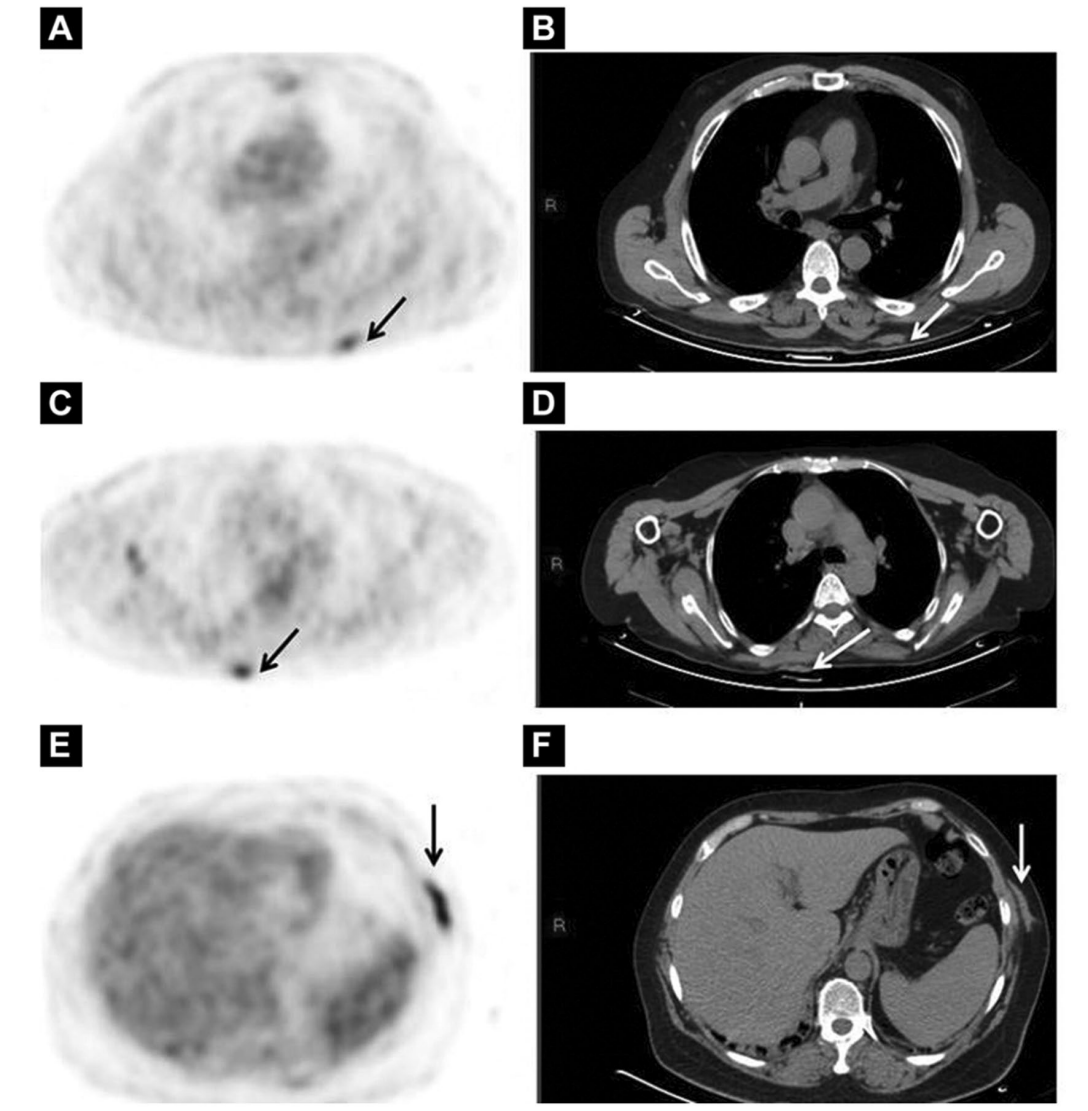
A patient with cutaneous marginal zone lymphoma, an indolent *B*-cell PCL. Subcutaneous lesions (white arrows) on axial CT (B, D, F) are visible but difficult to detect. These same lesions are more distinct and easier to identify on axial ^18^F-FDG PET/CT (A, C, E). This study provides evidence that ^18^F-FDG PET/CT may be superior to CT at detecting not only aggressive PCLs, but also indolent PCLs with subcutaneous presentation. Images courtesy of Davidson 2020 [19]
